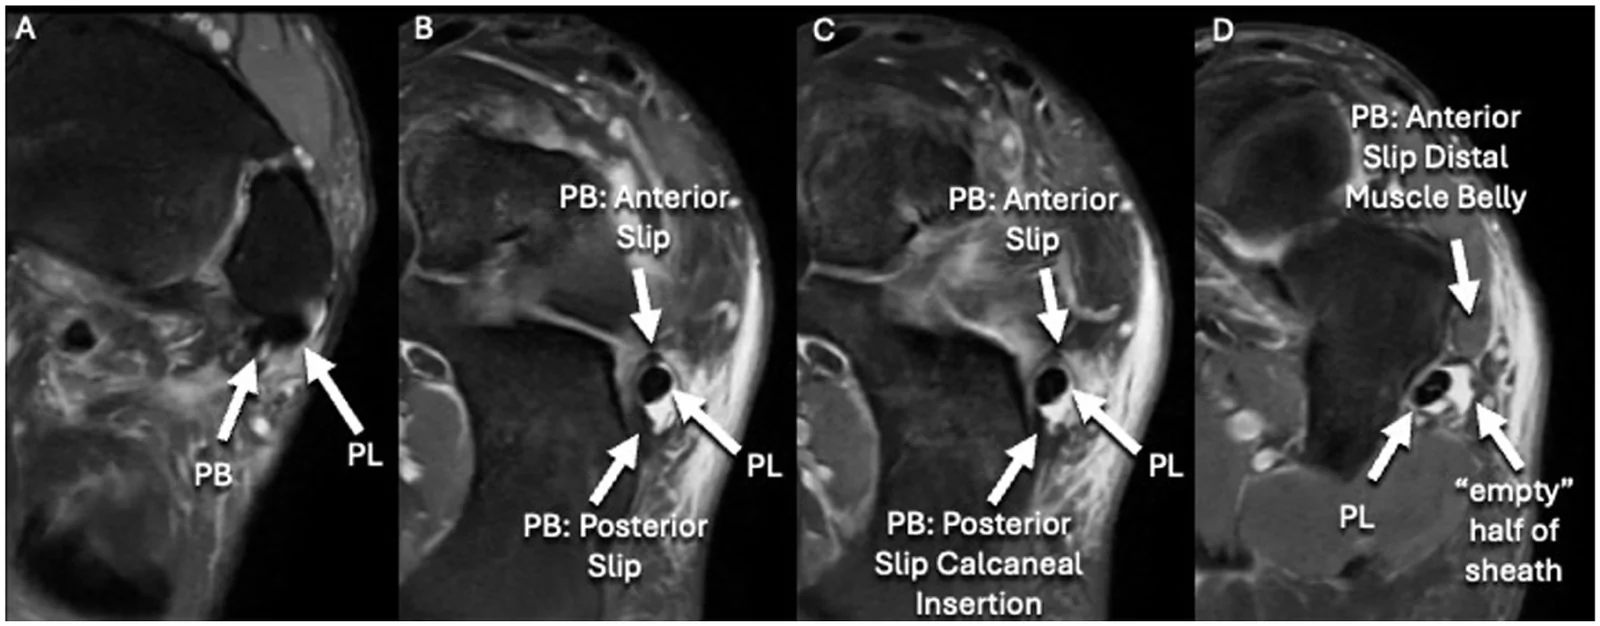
Axial oblique proton density fat-suppressed MRI images of the left ankle (A-D, proximal to distal). The diminutive peroneus brevis bifurcates proximal to the fibular tip into a posterior slip (inserting on the lateral calcaneus) and an anterior slip with a distinct distal muscle belly forming a digastric configuration. The peroneal tendon sheath is half-empty distally adjacent to the peroneus longus, where a normal-caliber peroneus brevis would be expected. PB, peroneus brevis; PL, peroneus longus.
